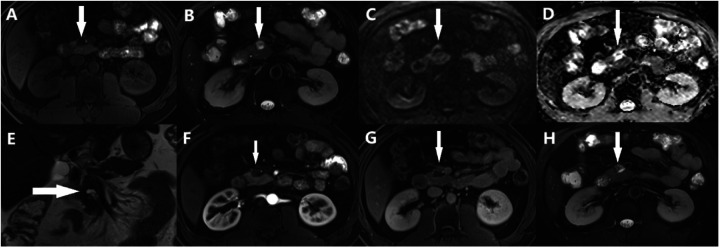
Abdominal MRI showed a solid lesion with cystic lesion, (A) T1WI showed hypointensity (arrows), (B) T2WI showed high-intensity (arrows), (C) DWI showed iso-intensity (arrows), (D) ADC showed high-intensity (arrows), (E) Coronal T2WI showed a ring-shaped low signal on T2WI at the periphery of the lesion (arrows), (F-H) the lesion had obvious progressive enhancement.
